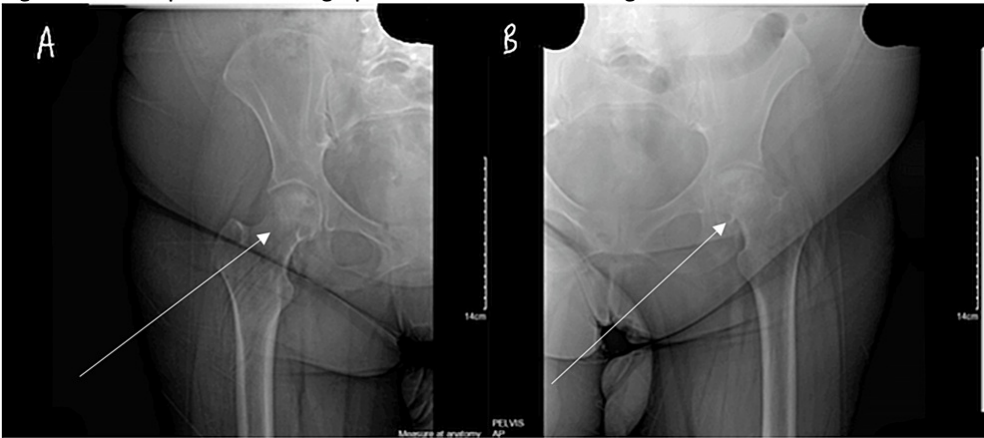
Anteroposterior radiographs of the left (A) and right hip (B). Arrows indicate the area of possible avascular necrosis.
